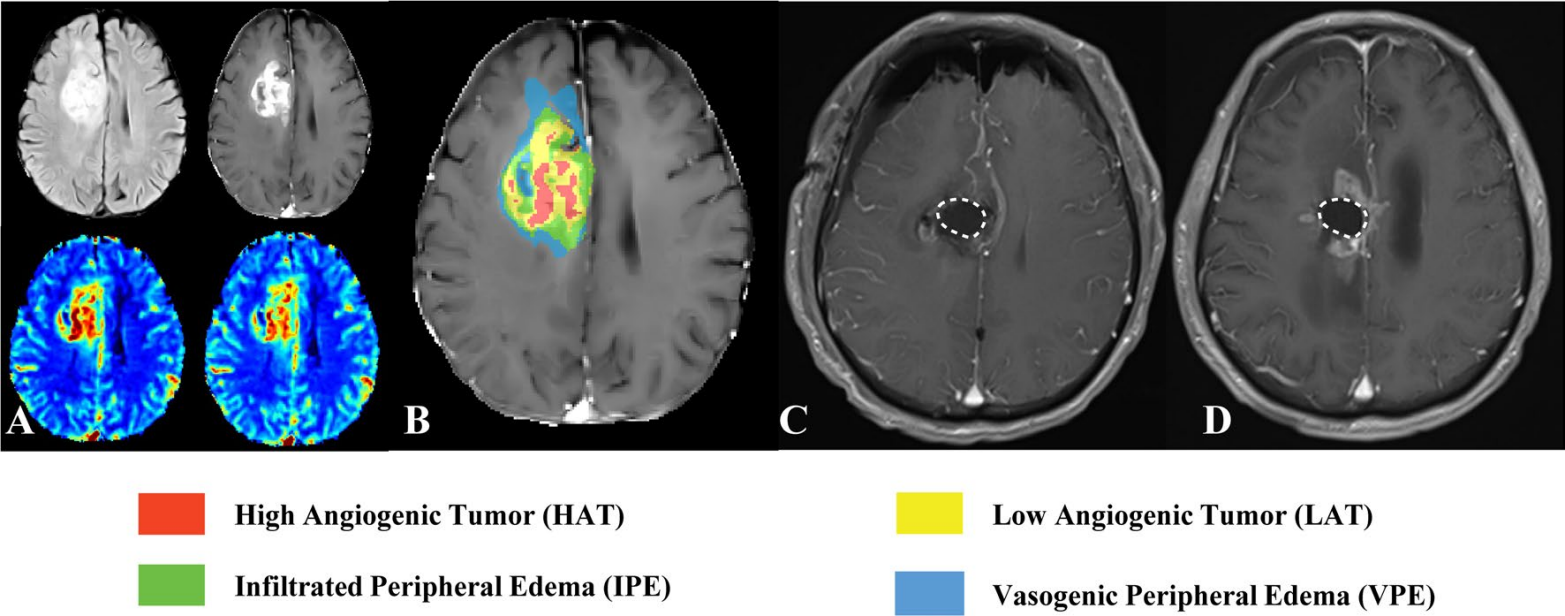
MRI scans in a 70-year-old man with glioblastoma, isocitrate dehydrogenase (IDH) wild-type, WHO grade IV, and tumor recurrence in the extra-resection cavity. Axial (A) fluid attenuation inversion recovery (FLAIR) image, contrast-enhanced T1-weighted image, cerebral blood volume (CBV) map, and cerebral blood flow (CBF) map are shown in small images (left to right, top to bottom). B Vascular habitat maps. The different colors indicate subregions of segmentation. C Twenty-four to 72 h postoperatively MRI. Contrast-enhanced T1-weighted image displayed gross total resection of the tumor and without enhancement of the surgical residual cavity (white dashed line). D Follow-up MRI. The tumor recurrence in the extra-resection cavity after 3 months
